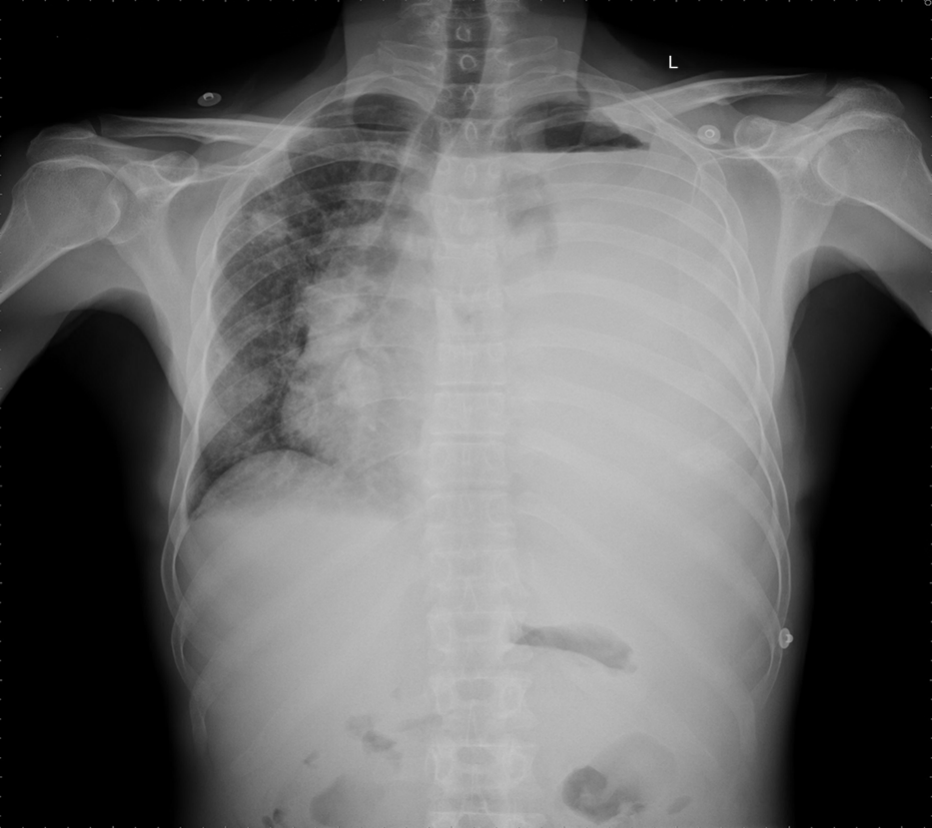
Chest X-ray showing massive left side pleural effusion and right upper zone nodular opacity (small air pocket in left apex is due to previous aspiration).
